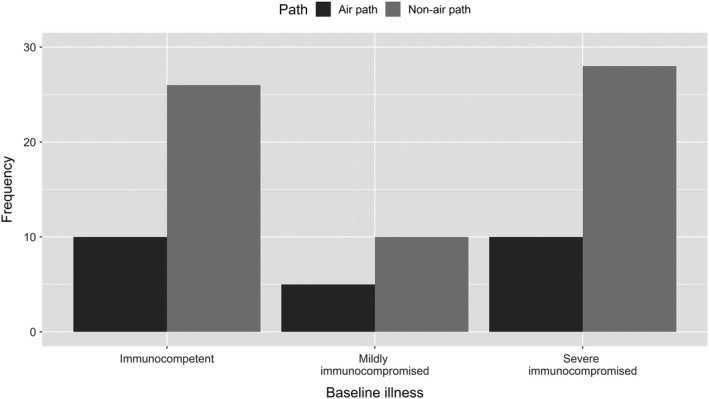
The features of distribution of PC lung lesions in CT imaging. CT, computerised tomography; PC, pulmonary cryptococcosis
